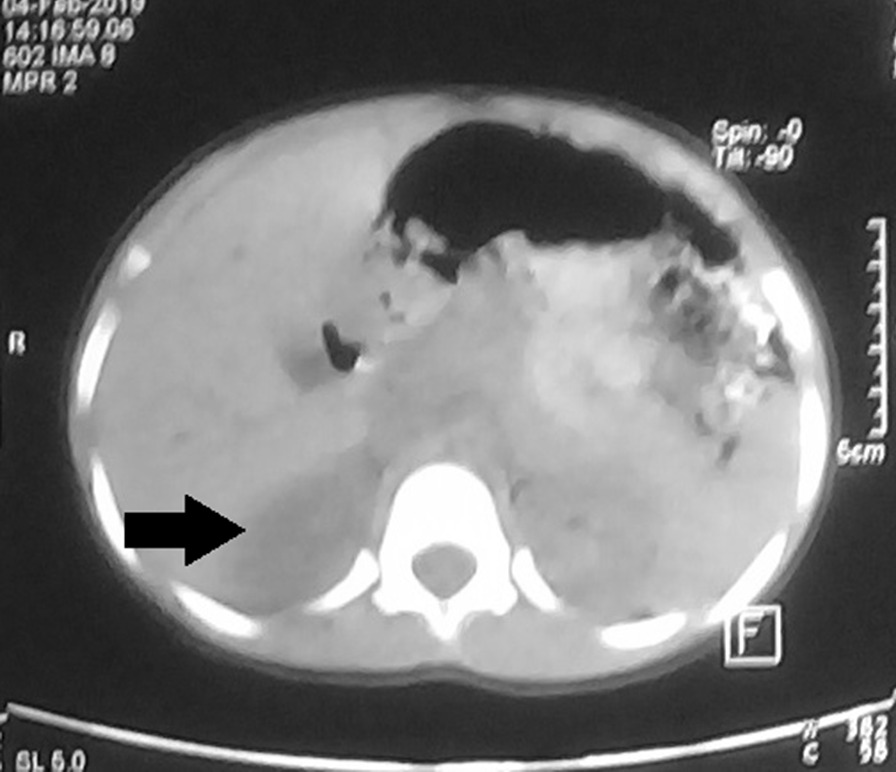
Abdominal computed tomography scan showing a right adrenal tumor (arrow)
